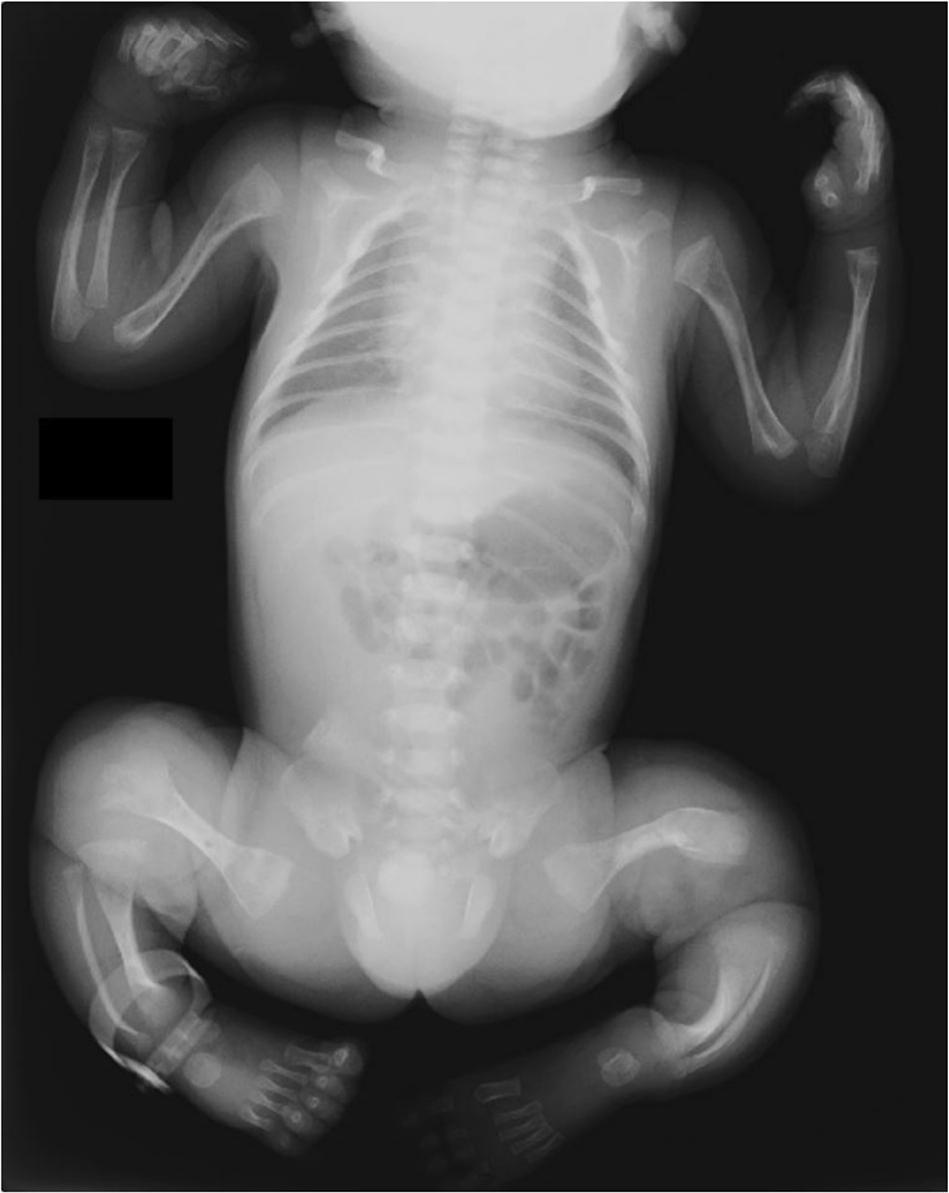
The neonatal X-ray showed deformity in the long bones, consistent with in utero fractures.
